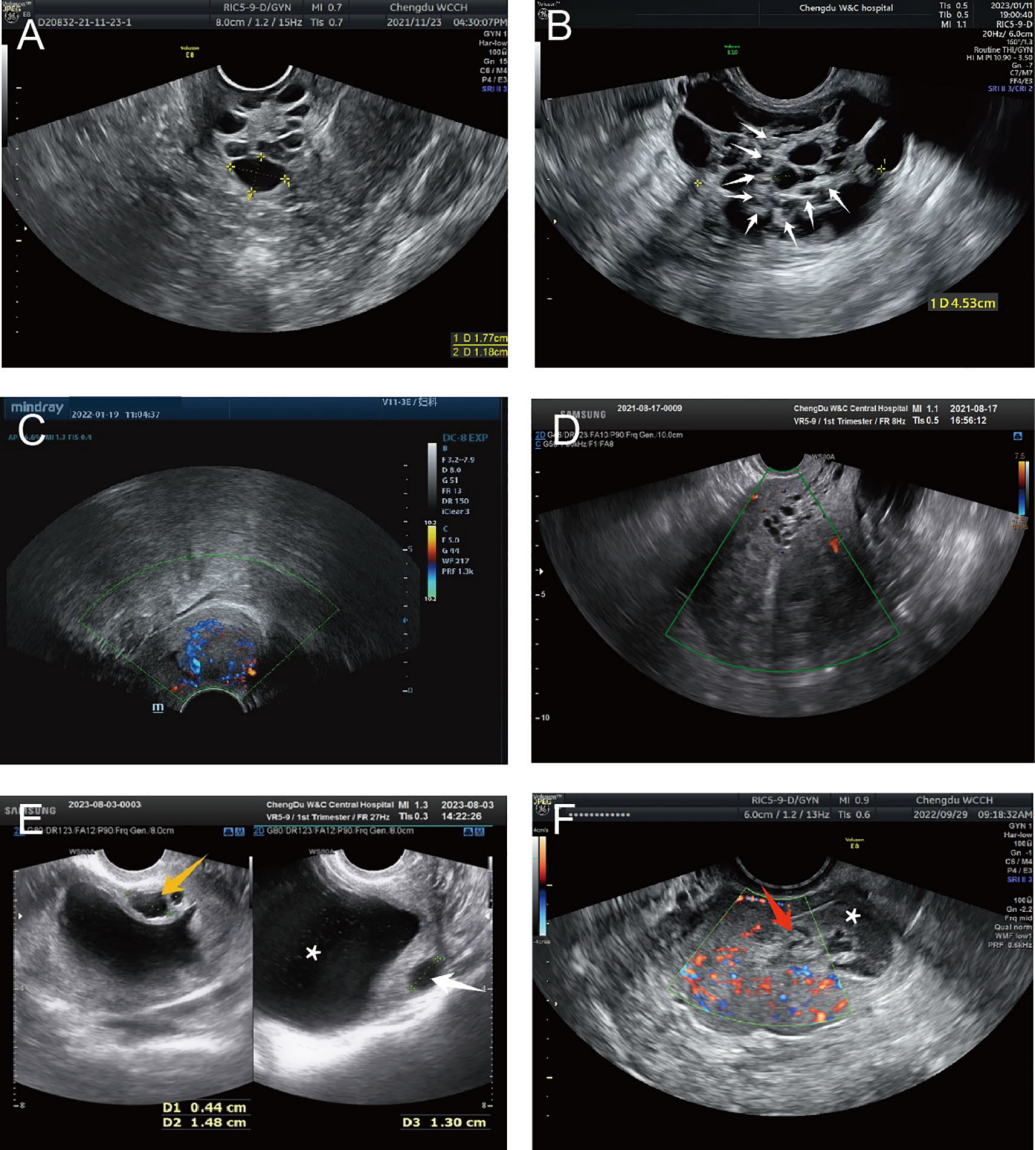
Representative ultrasound images of G-EAC. (A) Ultrasound image of type I G-EAC shows the multilocular cystic lesion in the cervix. (B) Ultrasound image of type II G-EAC demonstrates a multilocular cystic-solid mass, with smaller cysts surrounded by larger cysts, presenting a “cosmos sign.” Solid echoes (white arrows). (C) Type III G-EAC appears as a solid hypoechoic mass with poorly defined borders and no apparent capsule. CDFI exhibits abundant blood flow signals. (D) Cervical and uterine ultrasound images of case 6 shows vesicular echoes extending from the cervix to the lower uterine segment. (E) Cervical and uterine ultrasound images of case 10 demonstrates ECF and multiple cervical cystic echoes. Vesicular implants are observed within the myometrium. Multilocular cystic cavities in the cervix (orange arrows), intramural vesicular implants (white arrows), and ECF (asterisk). (F) Uterine CDFI of case 8 (axial plane). A slightly hyperechoic area is noted in the right uterine cornu with indistinct boundaries from the myometrium. Abundant punctate and linear blood flow signals are detected. Postoperative pathology confirms SMMN-FGT. Cornua uteri (red arrows) and ECF (asterisk). CDFI, color Doppler flow imaging; ECF, endometrial cavity fluid; G-EAC, gastric-type endocervical adenocarcinoma; SMMN-FGT, synchronous mucinous metaplasia and neoplasia of the female genital tract.
